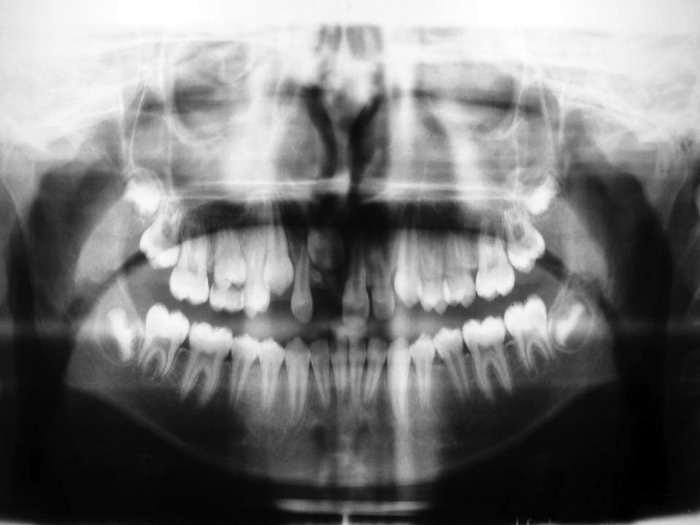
Orthopantomograph showing the presence of a
